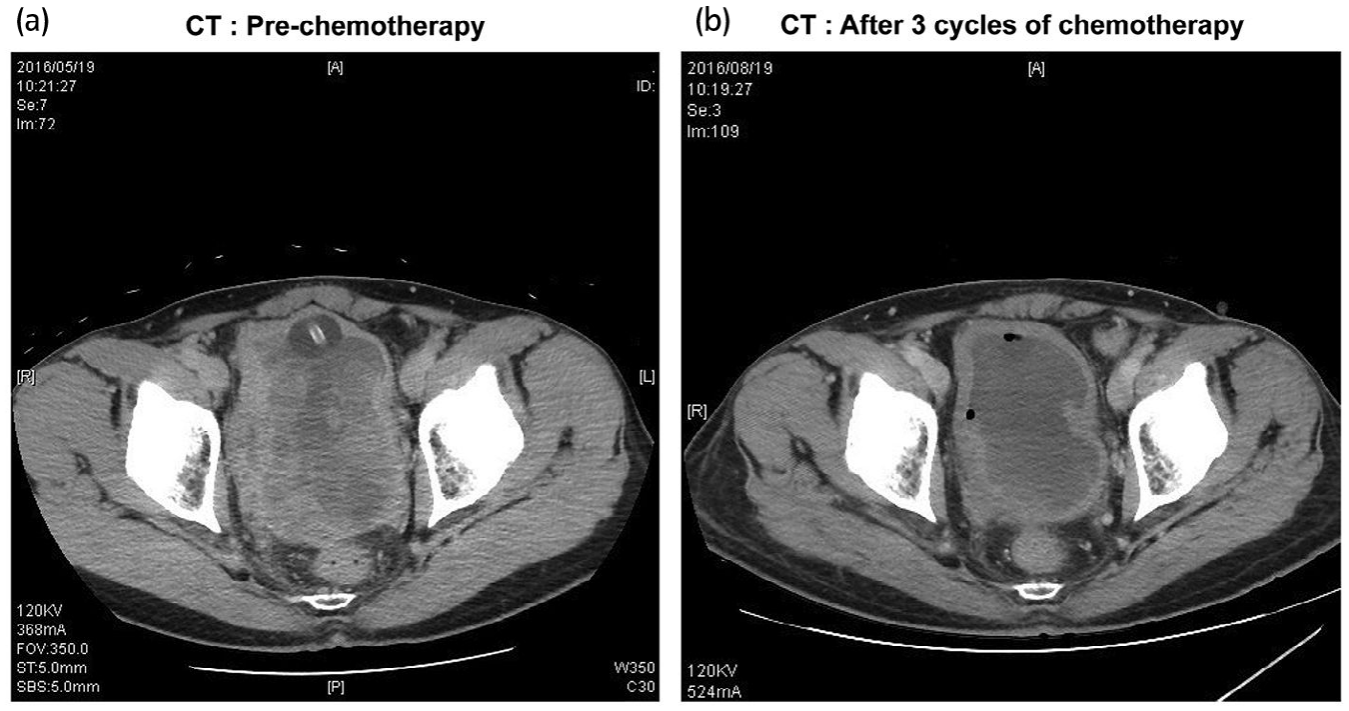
CT images (a) prechemotherapy and (b) after three cycles of neoadjuvant chemotherapy. After the three cycles of MAID regimen, the tumor shrank by 28% and necrotic changes were obvious.
